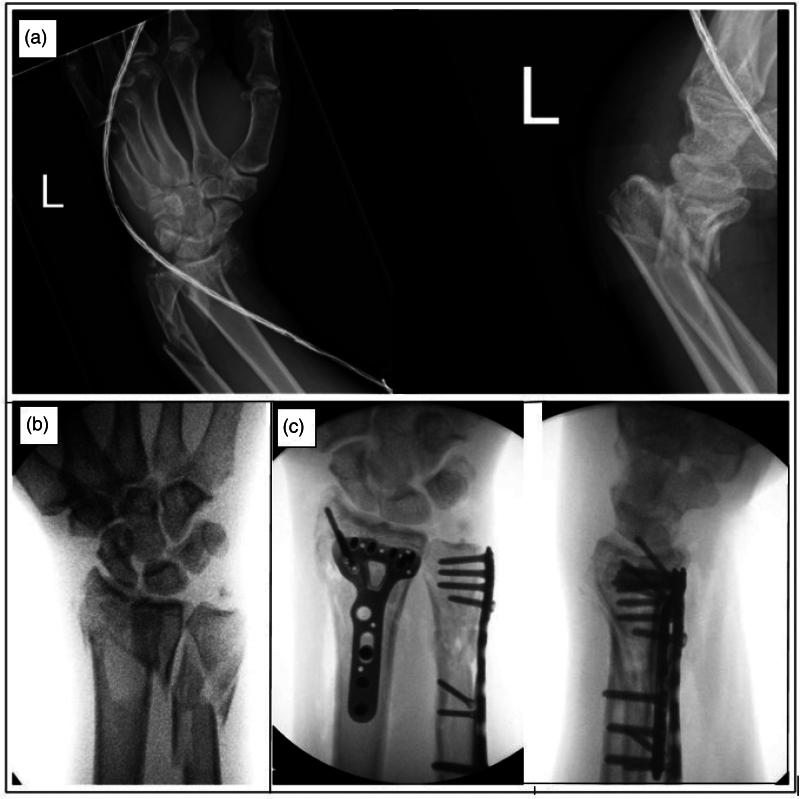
Radiographs of the wrist. (a) Anteroposterior and lateral views of the wrist at presentation. (b) Wrist in traction in wrist tower. (c) Lateral and anteroposterior views of the wrist on completion of surgery.
